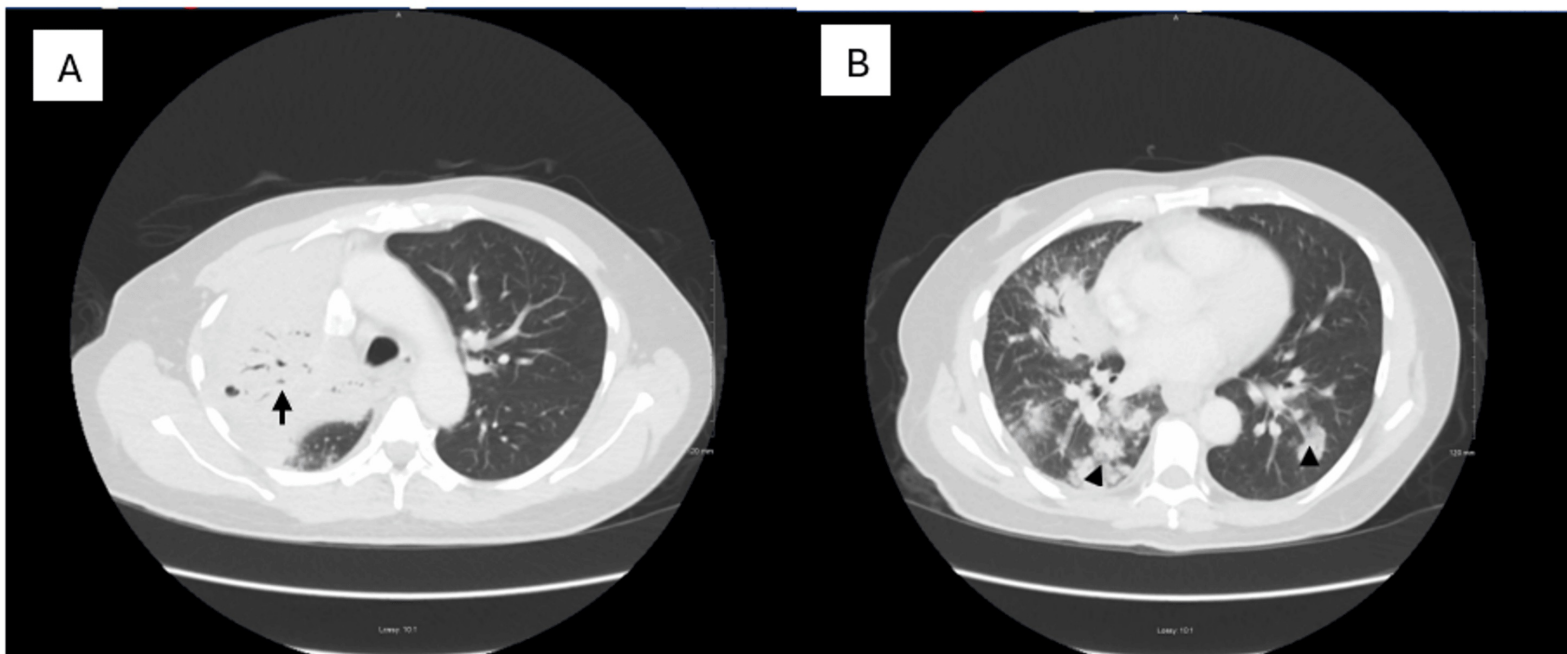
(A) Transverse chest CT image showing extensive consolidative changes in the right upper lobe (arrow) with mild mediastinal lymphadenopathy. (B) Patchy and nodular infiltrates are present in the bilateral lower lobes (arrowheads). These radiographic findings are consistent with pulmonary blastomycosis in the setting of systemic fungal infection.
